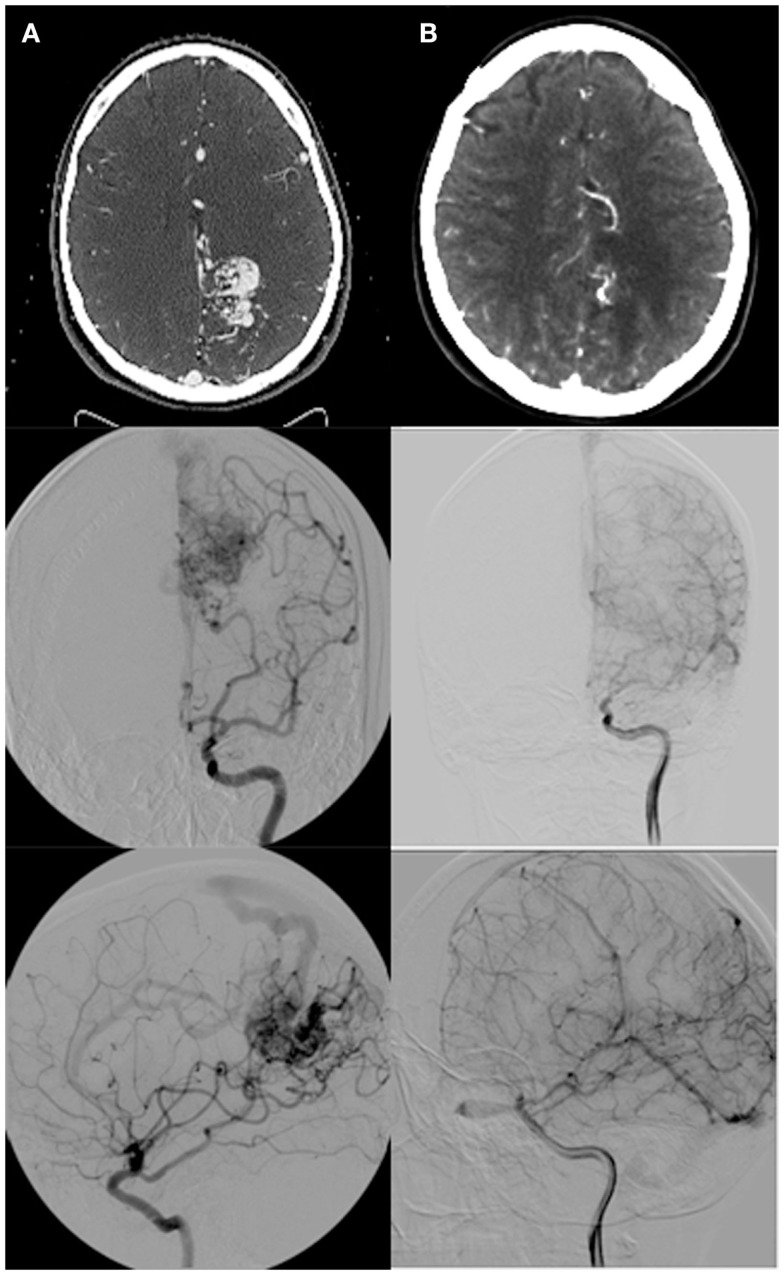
(A) Pre-radiosurgery, and (B) post-radiosurgery CT angios and angiograms for a representative case with follow-up images taken at 2 years post-radiosurgery demonstrating complete nidal obliteration with no residual draining vein.
